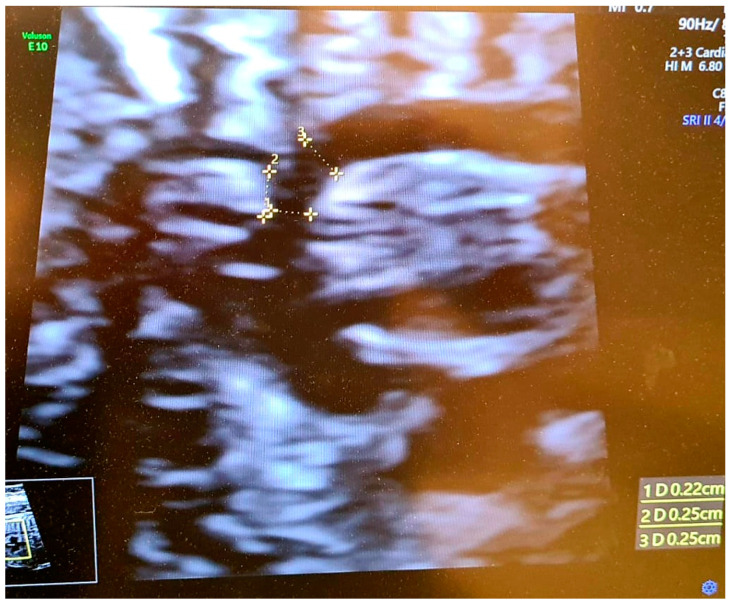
Ultrasound view: Correct visualization of the aortic arch at 23.5 week of pregnancy—with visible brachiocephalic trunk. The dimension from carotid distance to subclavian (2 D) = 2.5 mm, transverse aortic dimension (1 D) = 2.2 mm, calculated CSAi = 0.88. Aortic isthmus (3 D) 2.5 mm, z-score = −0.58.
